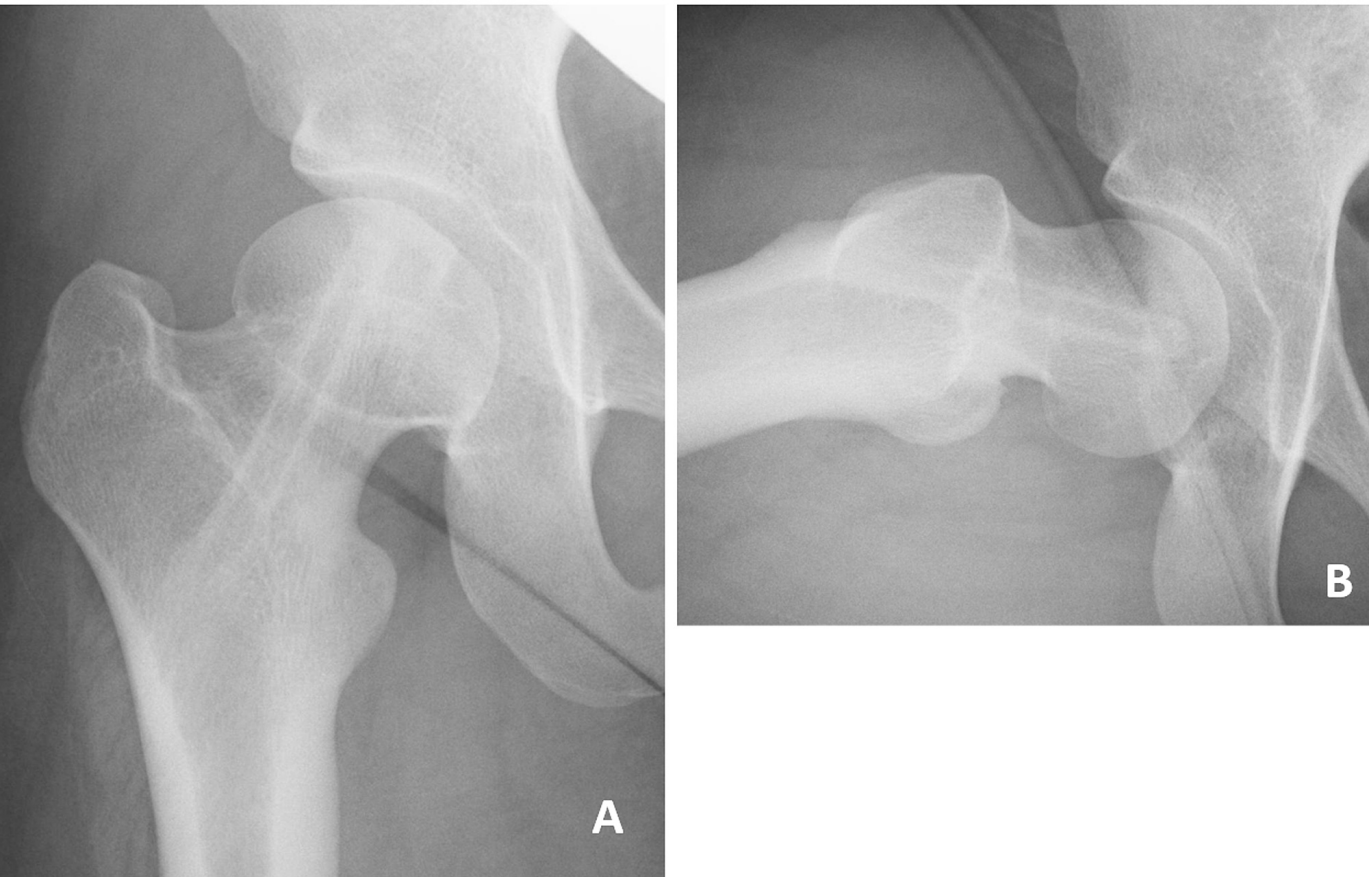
Imaging at last follow up (15 years old). (A) Anteroposterior and (B) axial radiographs of the right hip, at final follow up (end of her bone growth; 10 years after the first surgery).
